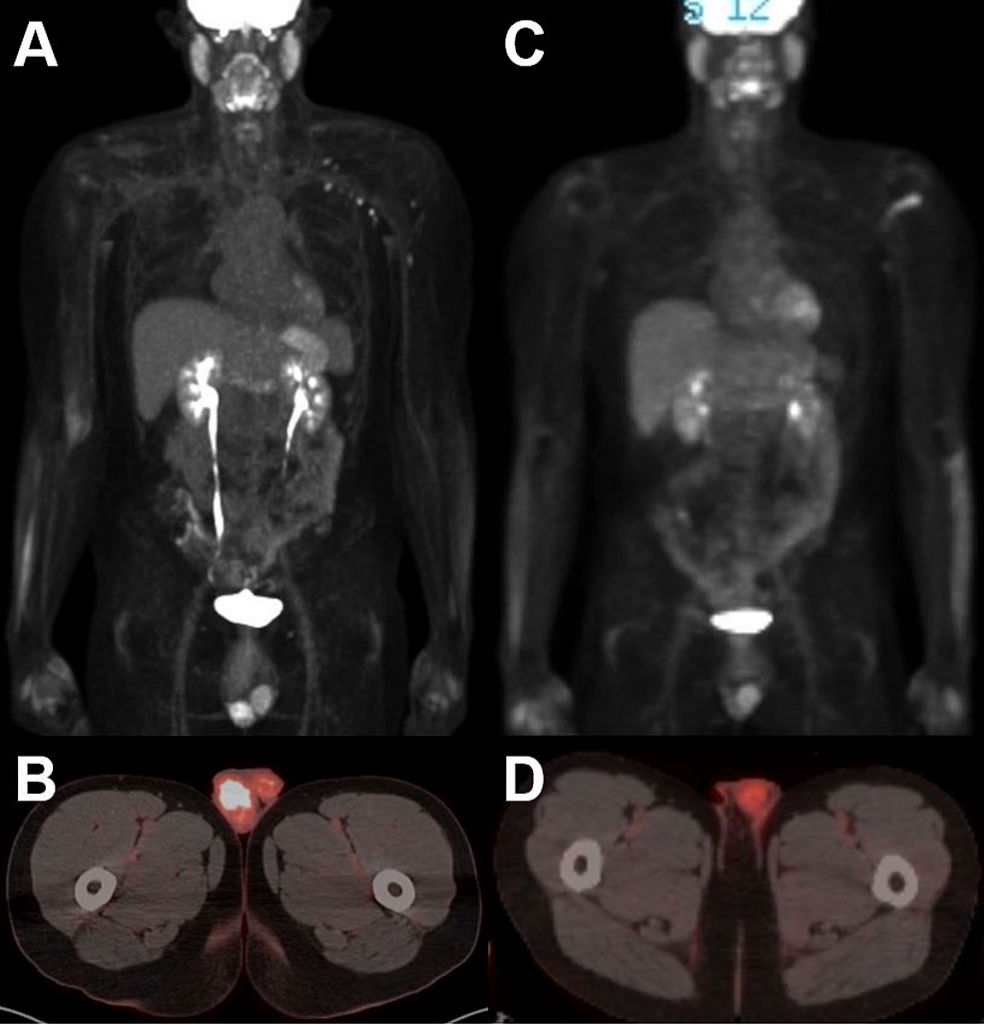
FDG PET-CT prior to orchiectomy and bridging radiation therapy (A and B), and after orchiectomy and bridging radiation therapy (C and D) FDG: fluorodeoxyglucose; PET: positron emission tomography; CT: computed tomography
